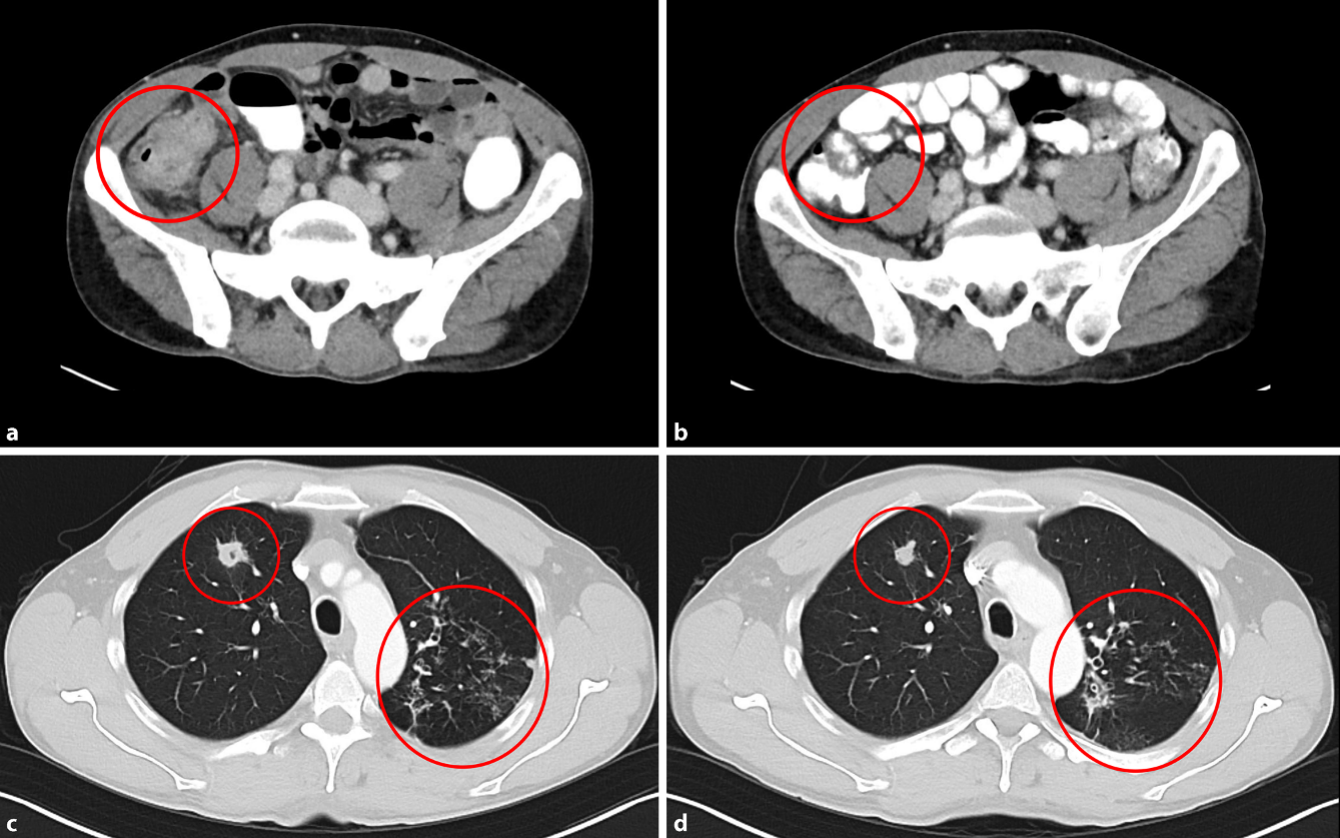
CT scan of the abdomen and the chest showing a gastrointestinal tumor mass in the cecum (*red circle*) and a pulmonary nodule in the left upper lung lobe as well as infiltrates in the right upper lung lobe (*red circles*) at time of diagnosis (a, c) and after 3 months of antimycobacterial treatment, respectively (b, d)
